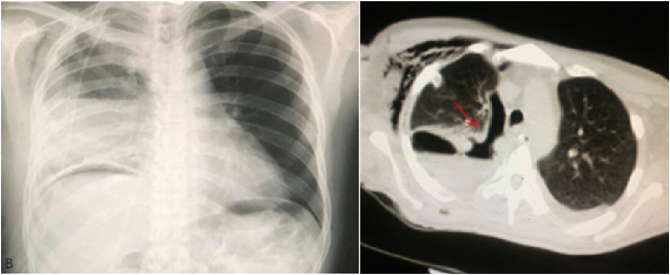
Which shows: the CXR on the left with the cavity and sub diaphragmatic free gas which was iatrogenic from laparotomy, and the CT-scan on the right with the cavity with air-fluid level (red arrows).
